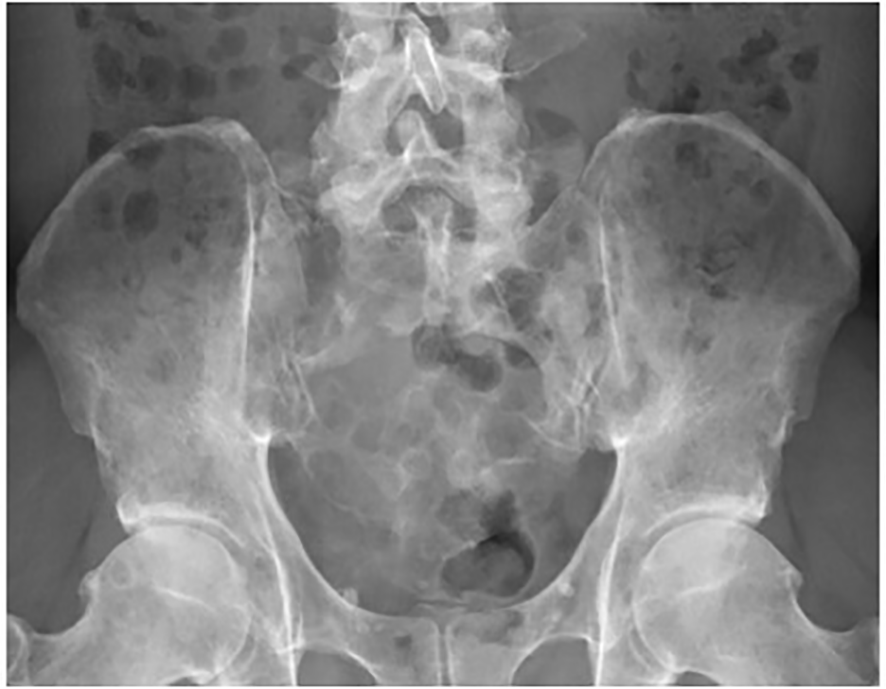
A 46-year-old male with extensive destruction of the sacrum and bilateral iliac bones secondary to multiple myeloma. Preoperative radiograph.
